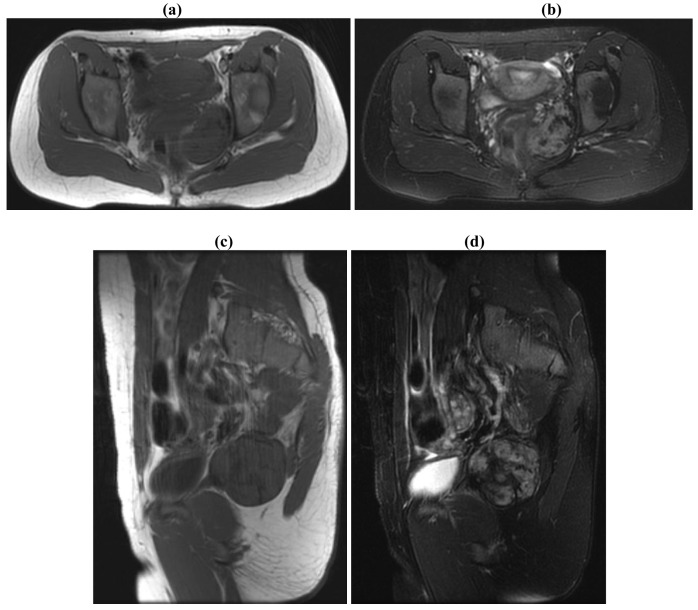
31/F with deep pelvic desmoid with a history of FAP treated with excision utilizing a combined Pfannenstiel and ischiorectal approach. Adjuvant radiation was administered as a result of positive margins. MRI Findings: There is an 8.4 × 6.8 × 5.5 cm enhancing irregularly-shaped mass centered in the left ischiorectal fossa. There is full-thickness invasion of the left obturator internus muscle. The mass extends across the midline to the right with invasion of the perirectal muscles as well as posteriorly up to, and likely involving a small portion of the gluteus maximus muscle on the left. (a) Axial T1; (b) Axial T2; (c) Sagittal T1; (d) Sagittal T2.
